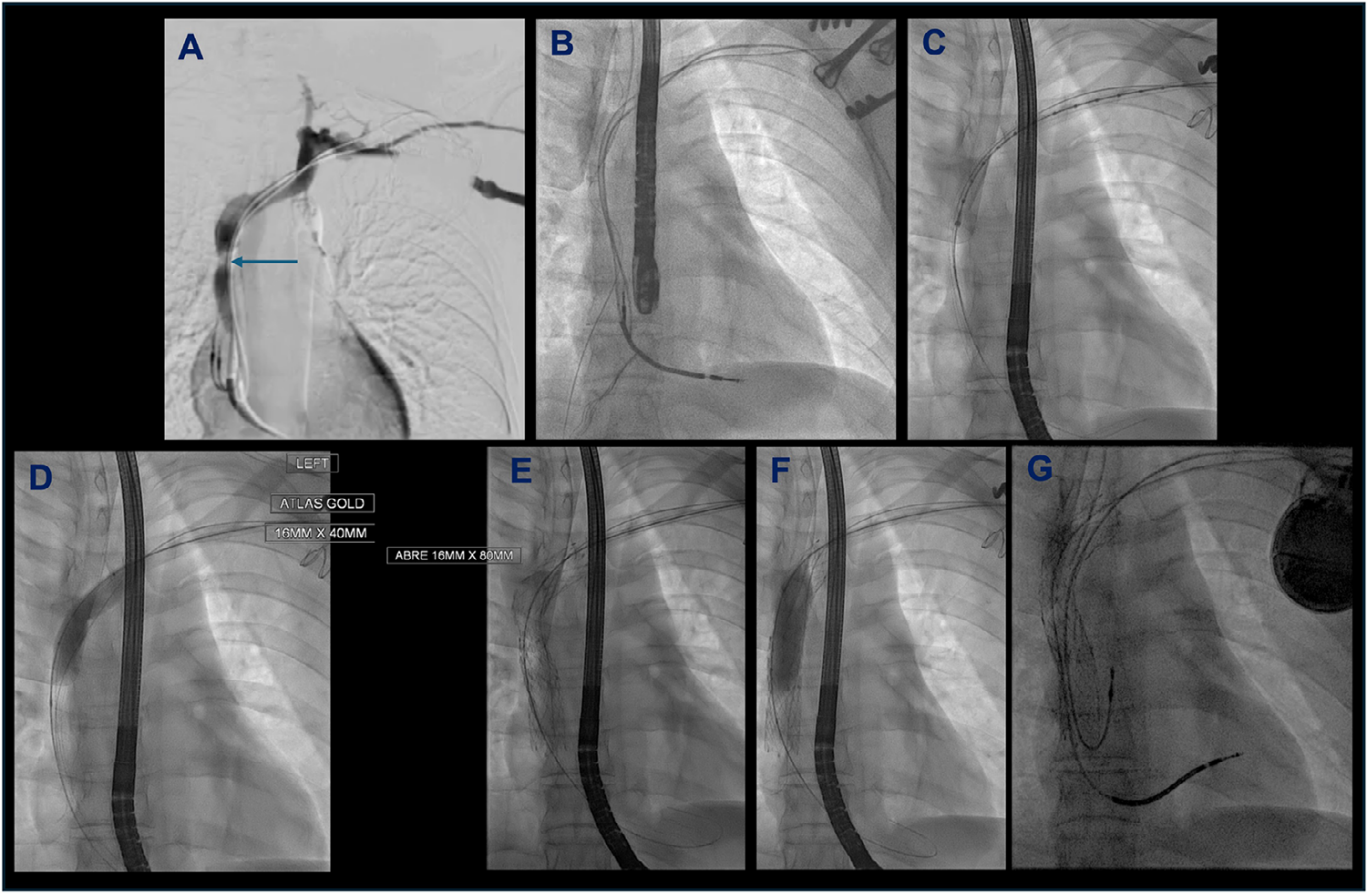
Workflow of case for extraction, venous stenting, and re‐implantation. (A) Digital subtraction angiography using contrast injection from left arm demonstrating SVC occlusion (arrow). Note also partial azygous filling; (B) baseline fluoroscopy; (C) following lead extraction, intravascular ultrasound performed to characterize lesion; (D) initial balloon venoplasty; (E) stent deployment; (F) stent post‐dilatation; (G) final image with transvenous leads re‐implanted. [Colour figure can be viewed at wileyonlinelibrary.com]
